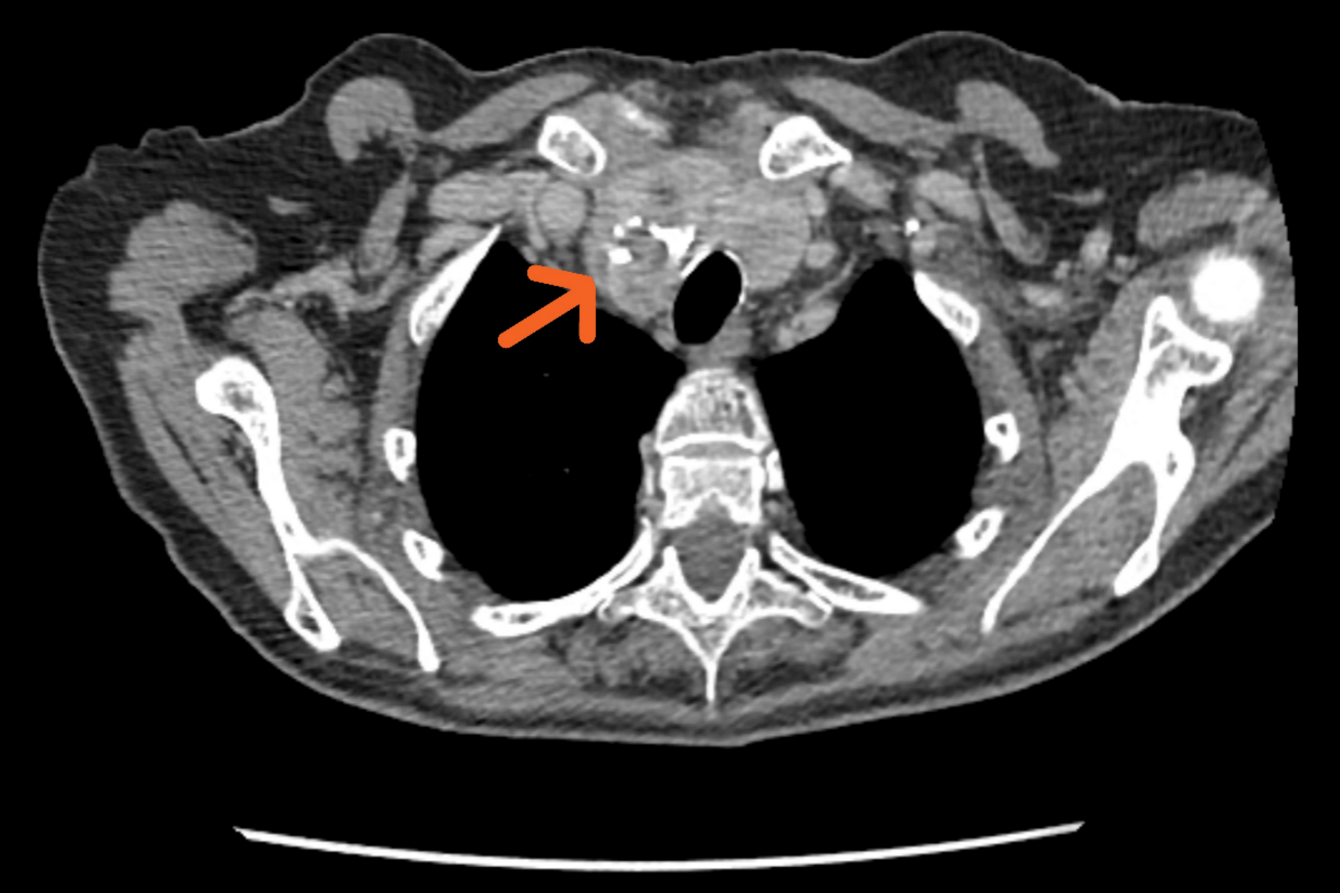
Axial CT scan of the neck CT: computed tomography
